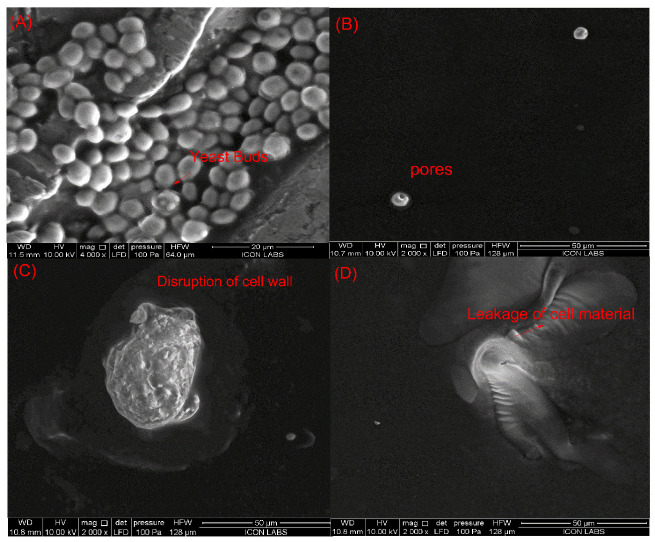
Influence of individual and sequential treatments on the morphology of the *Saccharomyces cerevisiae* (A) untreated sample; (B) pulsed light sample (0.84 J/cm^2^); (C) ultrasound sample (0.4 W/cm^3^); (D) PL + US (0.60 J/cm^2^ + 0.4 W/cm^3^).
